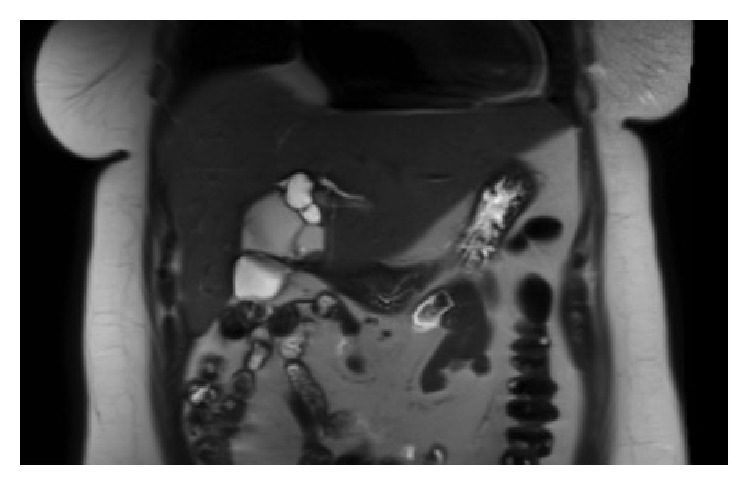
MRI picture showing septated biliary cystadenoma with nodule.
